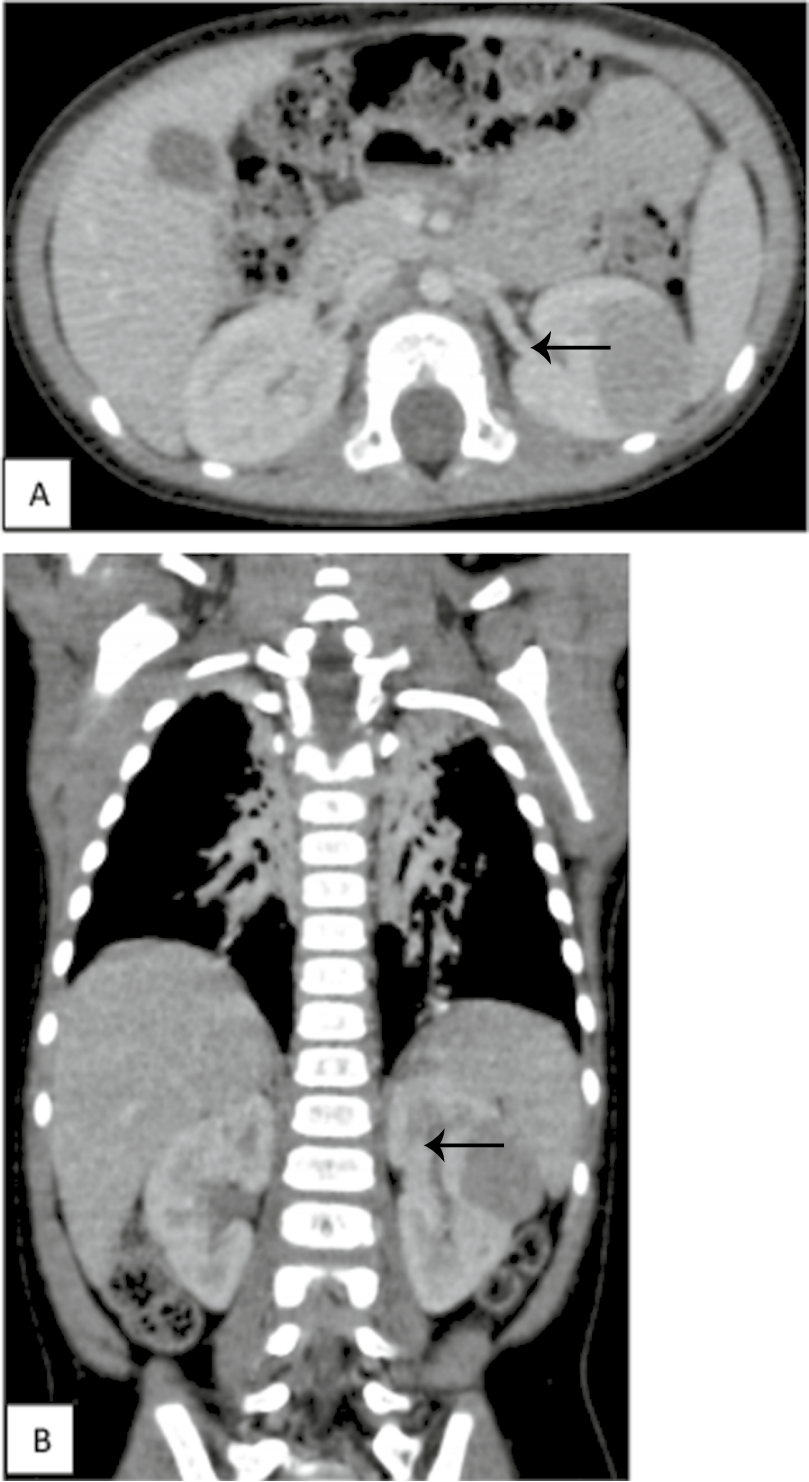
Abdominal CT scan in axial section (A) and coronal reconstruction (B) at portal time showing a tissue mass in the middle part of the left kidney with regular contours and which is enhanced homogeneously (← )
